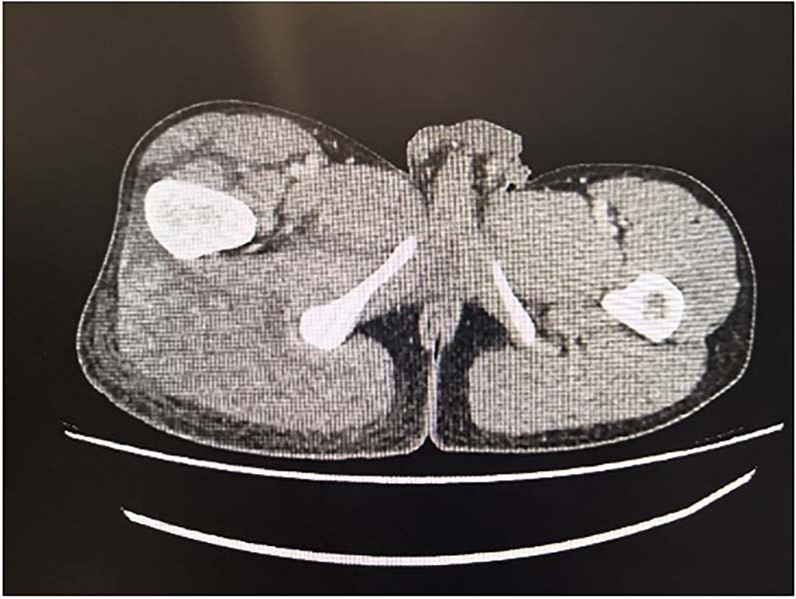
CT pelvis demonstrating asymmetric expansion of the right gluteus maximum muscle and associated oedema/stranding in keeping with muscle oedema. Fluid seen tracking into the posterior and lateral compartments of the thigh.
